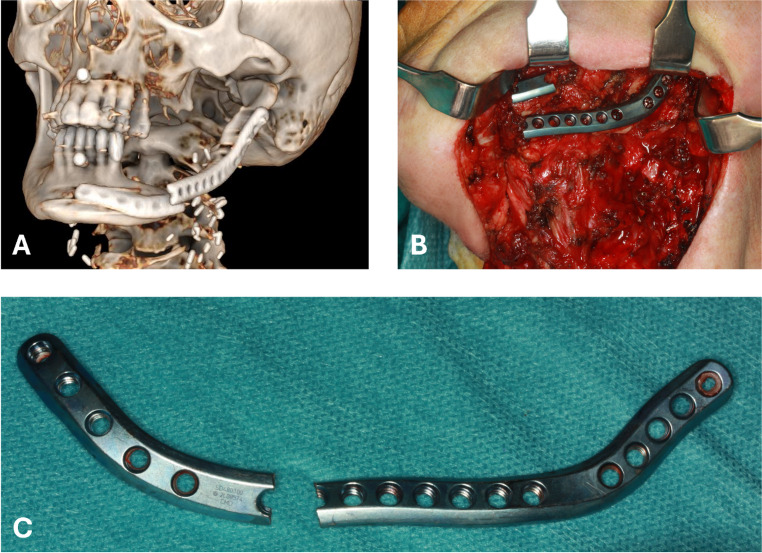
Fractured PSI in virtual reconstruction of a CBCT (A), intraoperative pictures in situ (B), and after explantation (C)
